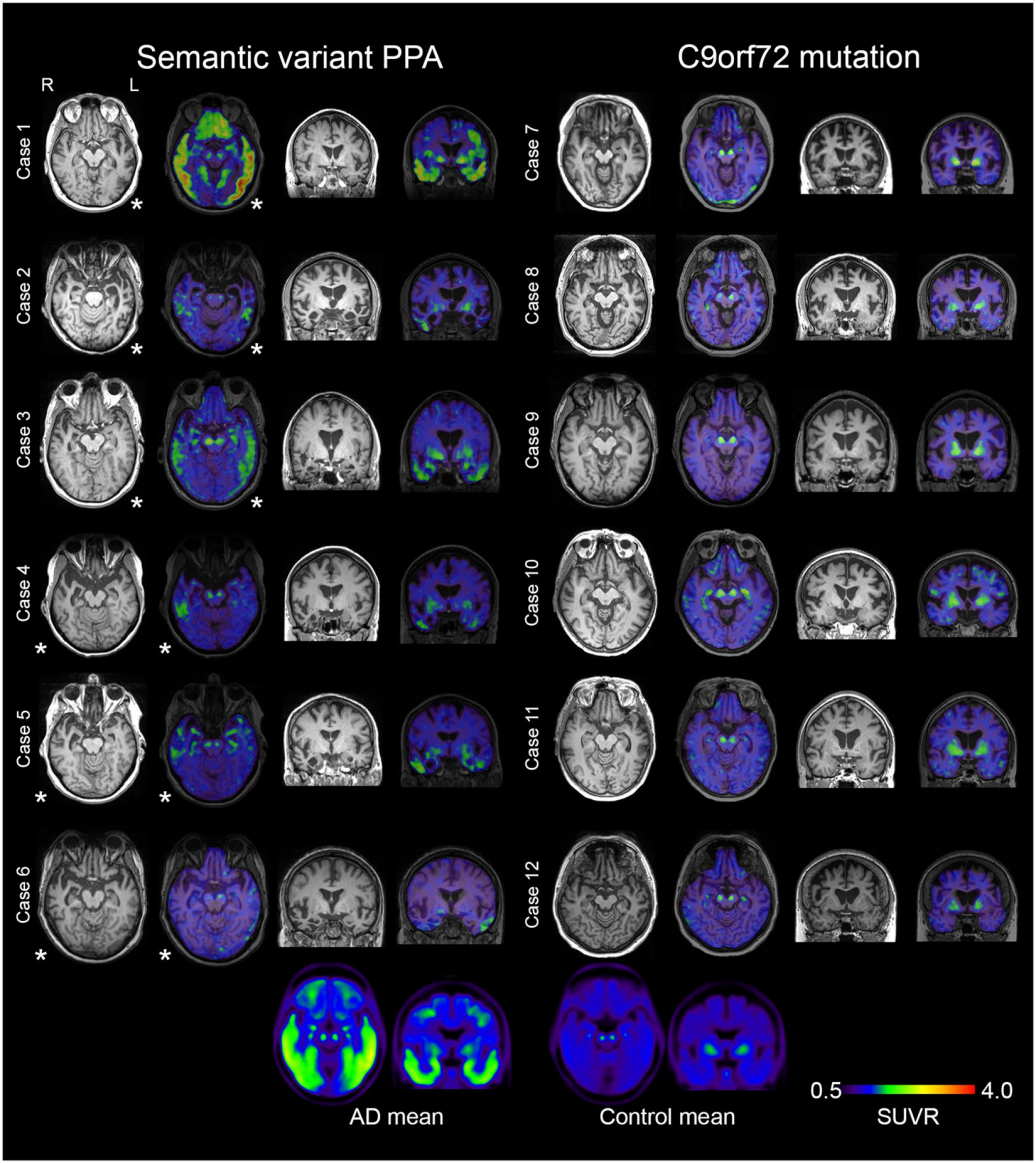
MRI and ^18^F-Flortaucipir PET scans in svPPA and *C9orf72*-mutation carriers. Transversal and coronal slices of structural (T1-mprage) MRI scans and ^18^F-Flortaucipir PET scans are shown for all included subjects with semantic variant primary progressive aphasia (svPPA) and bvFTD due to a hexanucleotide expansion in the *C9orf72*-gene. Case numbers correspond to case numbers in Table 1. Below the individual images are mean SUVR-images of 40 AD patients and the 54 controls in MNI-space, included for comparative purposes. The images are presented in radiological orientation (left in the image corresponds to patient right). Asterisks indicate the most affected side in the svPPA cases. Scale bar for PET-scans represents standardized uptake value ratios (SUVR). An identical figure with SUVR range 0.5–3 is included as Supplementary Fig. 5.
